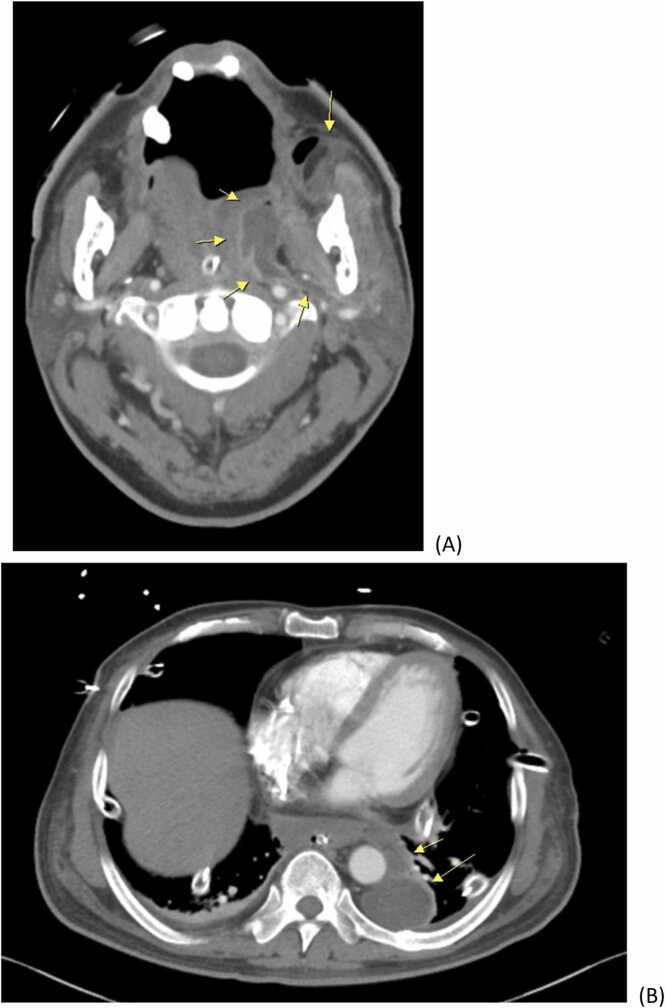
The repeated computed tomography scan was reported as below. Mild regression of residual abscess along left masseter muscle, submandibular region, palatine tonsil (yellow arrow), and mediastinum (A). Mild progressive bilateral lobulated complicated pleura effusion (yellow arrow) even adequate bilateral chest tube tips drainage function (B).
